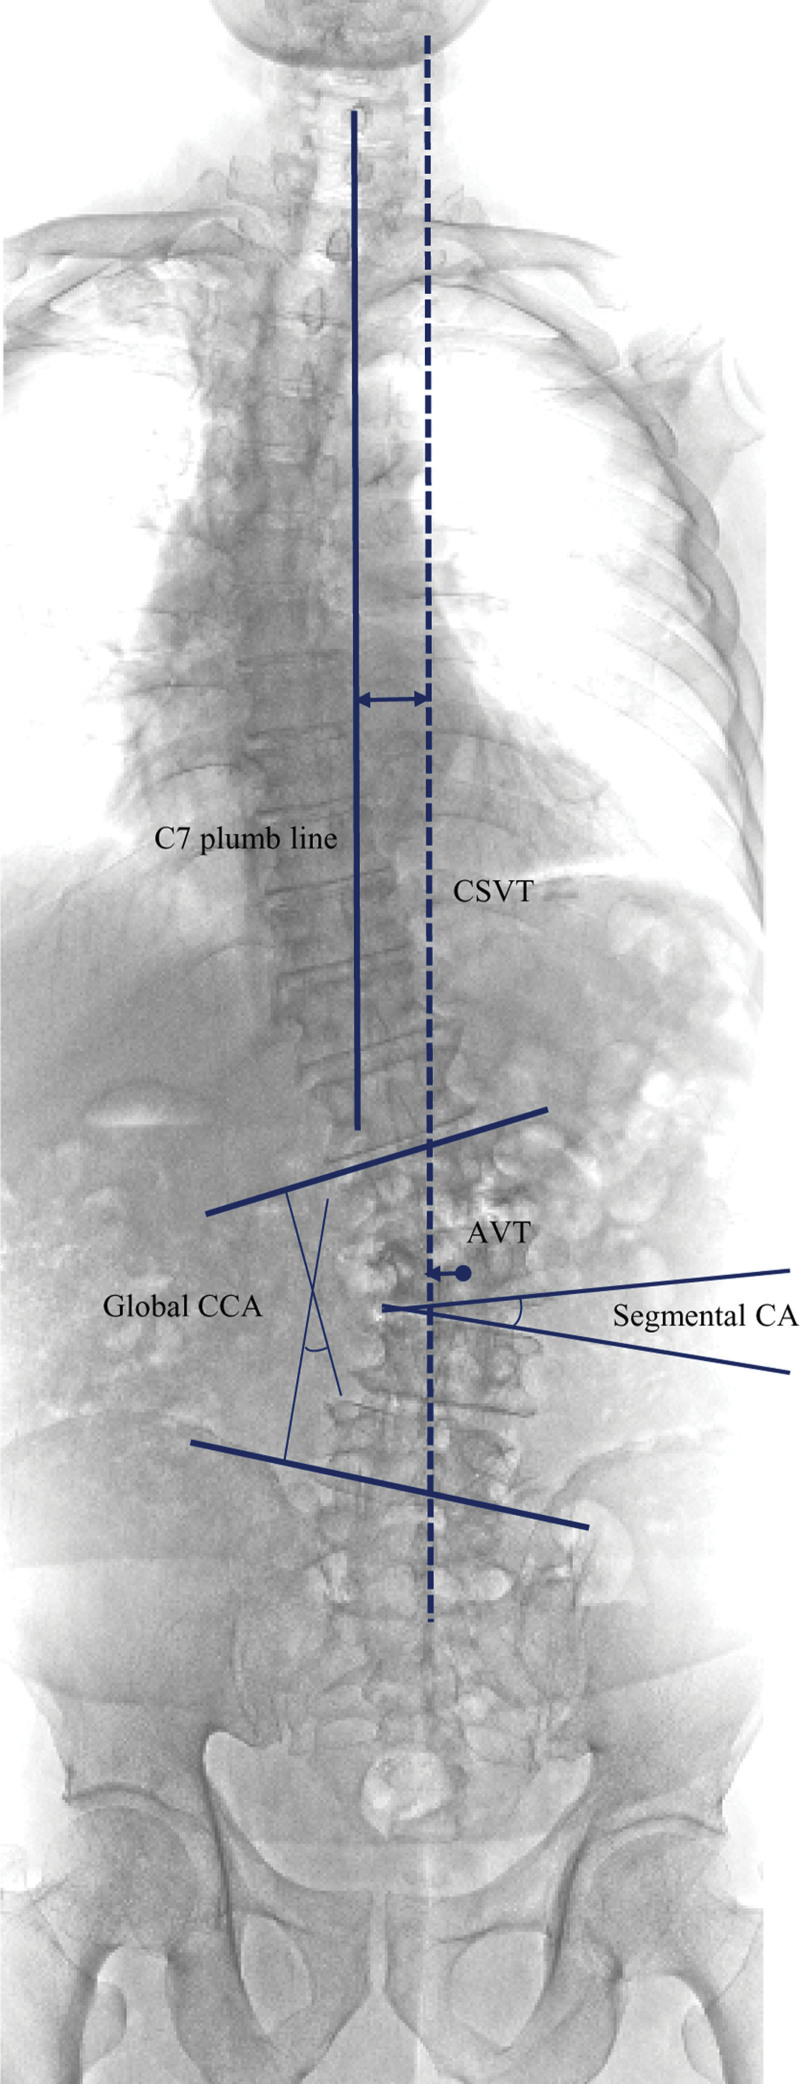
Measurement of radiologic parameters including global CCA, C7-CSVL, segmental CA, AVT in 36-inch standing anteroposterior radiographs. CCA: coronal Cobb angle, CSVL: central sacral vertical line, AVT: apical vertebral traslation, Dotted line indicated the CSVL.
